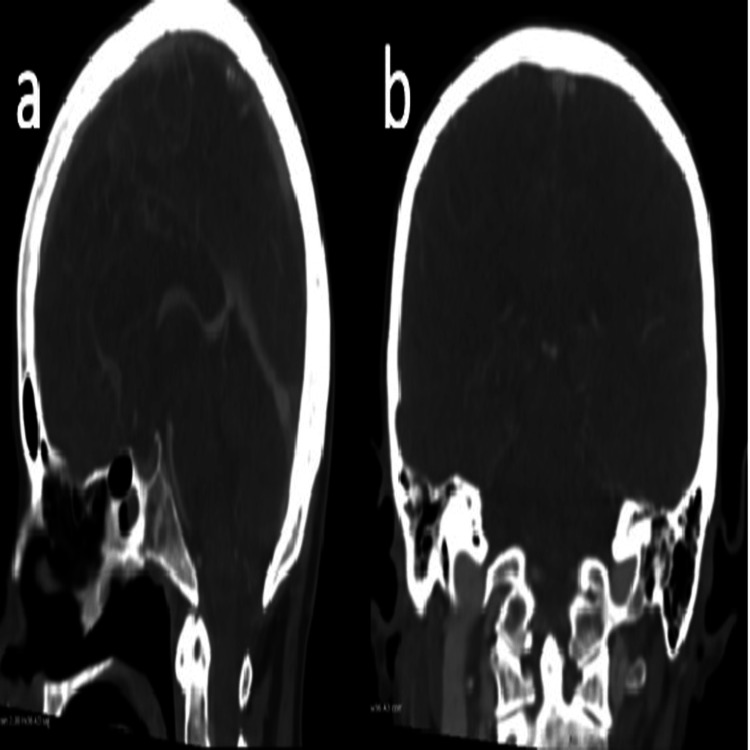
CT venogram of the head showing normal intracranial venous vasculature with no evidence of venous sinus thrombosis. (a) Sagittal view and (b) coronal view.
